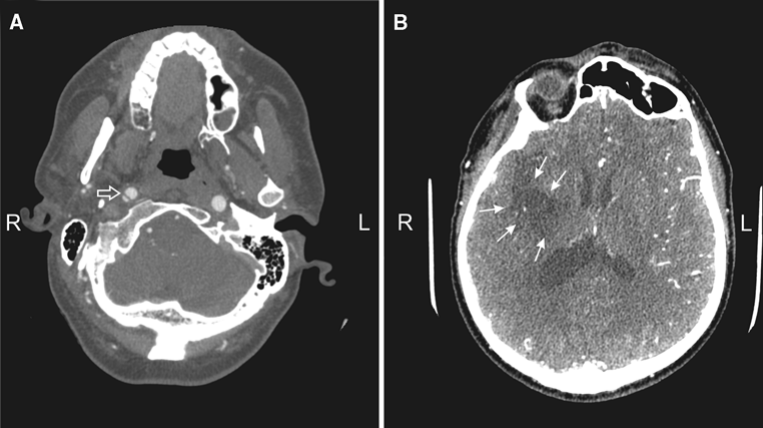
Computer tomography (CT) of the neck and head. a Segmental dissections of the right internal carotid artery. b Infraction involving deep structures of the right hemisphere including thalamus, genu and posterior limb of internal capsule, which on this single scan represent a part of the ischemic area
